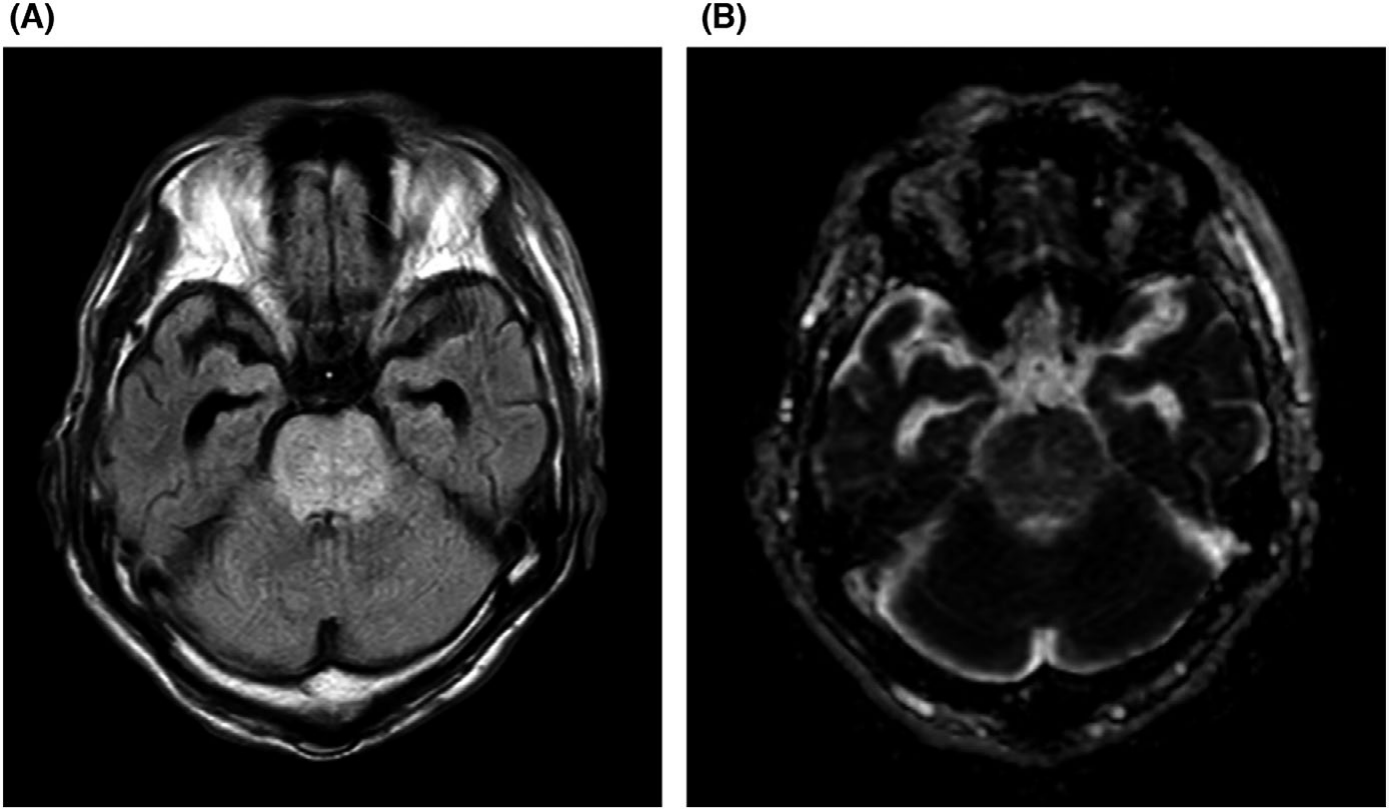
The magnetic resonance image (MRI) finding reveals a high‐intensity area at the pons with fluid‐attenuated inversion recovery (FLAIR) imaging and apparent diffusion coefficient (ADC) map (A and B), but isointensity of diffusion‐weighted imaging (DWI) and no evidence of the blood flow interruption. Both cerebellum and cerebrum were free of abnormal findings, and these findings suggest symmetric vasogenic edema at isolated pons. The characteristic of isolated brainstem PRES is that neurological symptoms are relatively mild for the abnormal imaging findings
